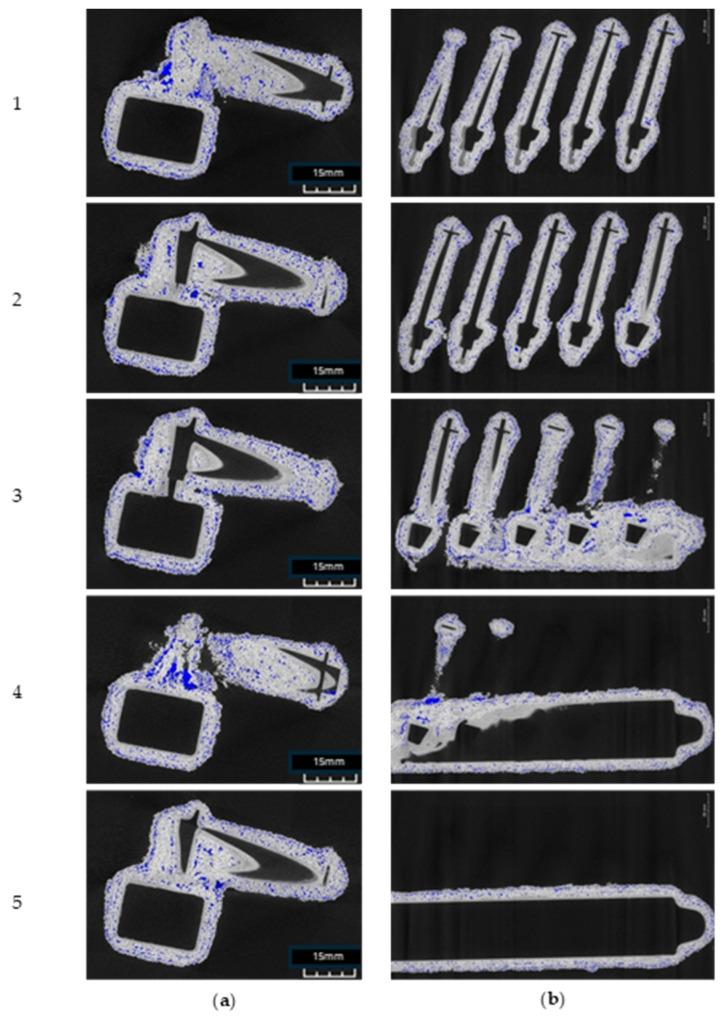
Cross-sectional X-ray tomographic images of the FM2 casting mould in planes (a) parallel and (b) perpendicular to the mould detail.
